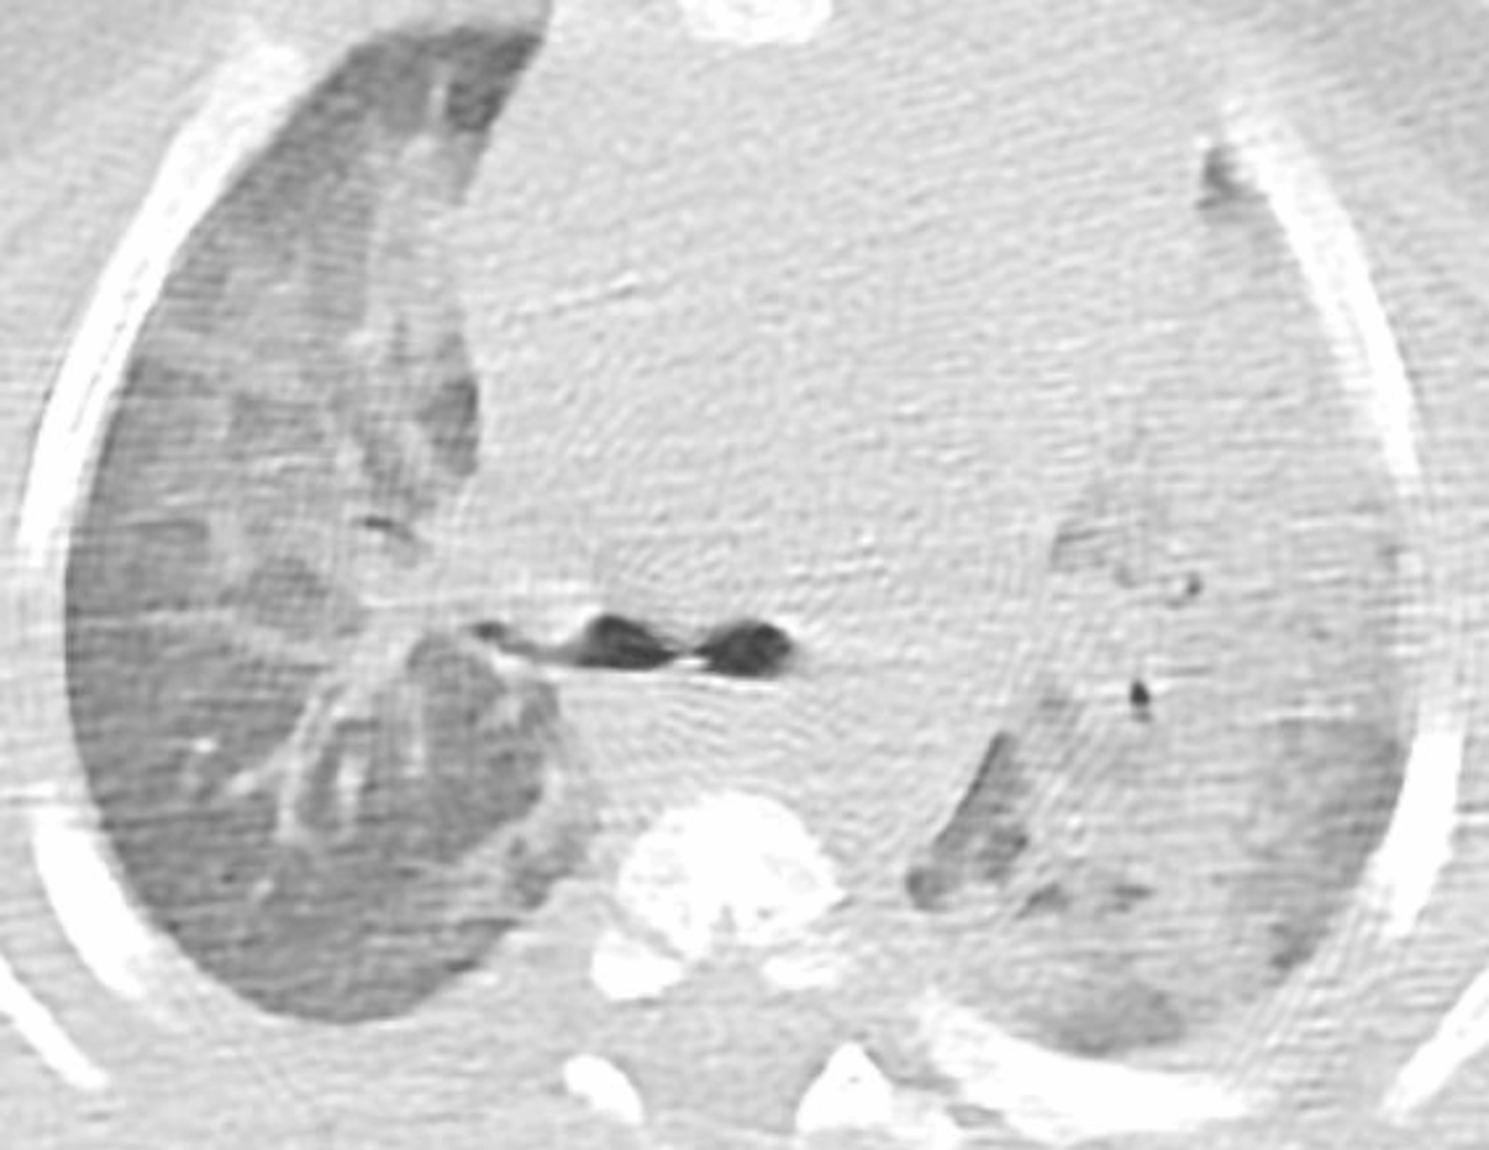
CT at the level of carina
